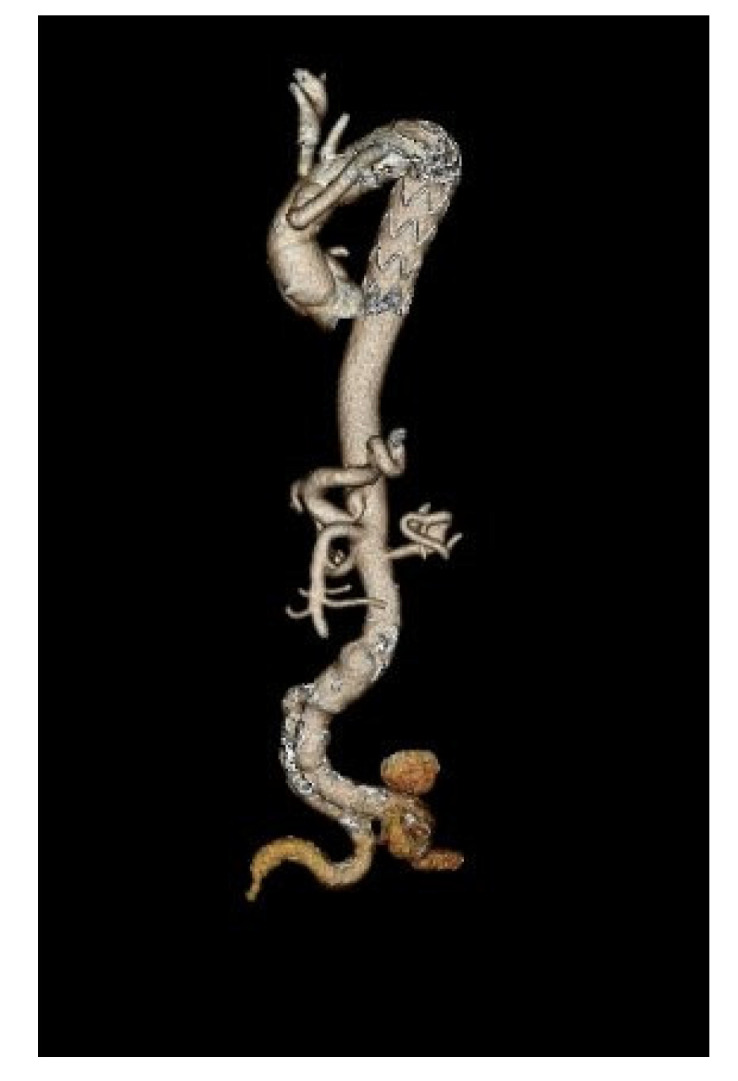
Post-operative computed tomography with 3D reconstruction after Thoraflex placement.
